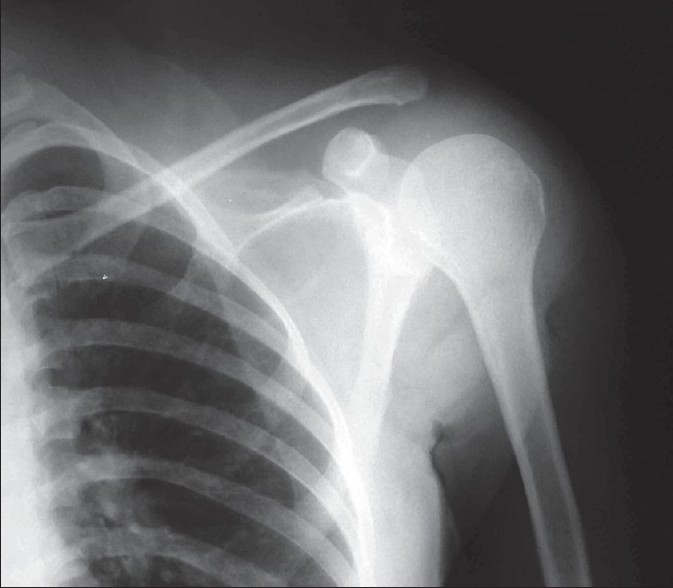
Postoperative x-ray of the patient
